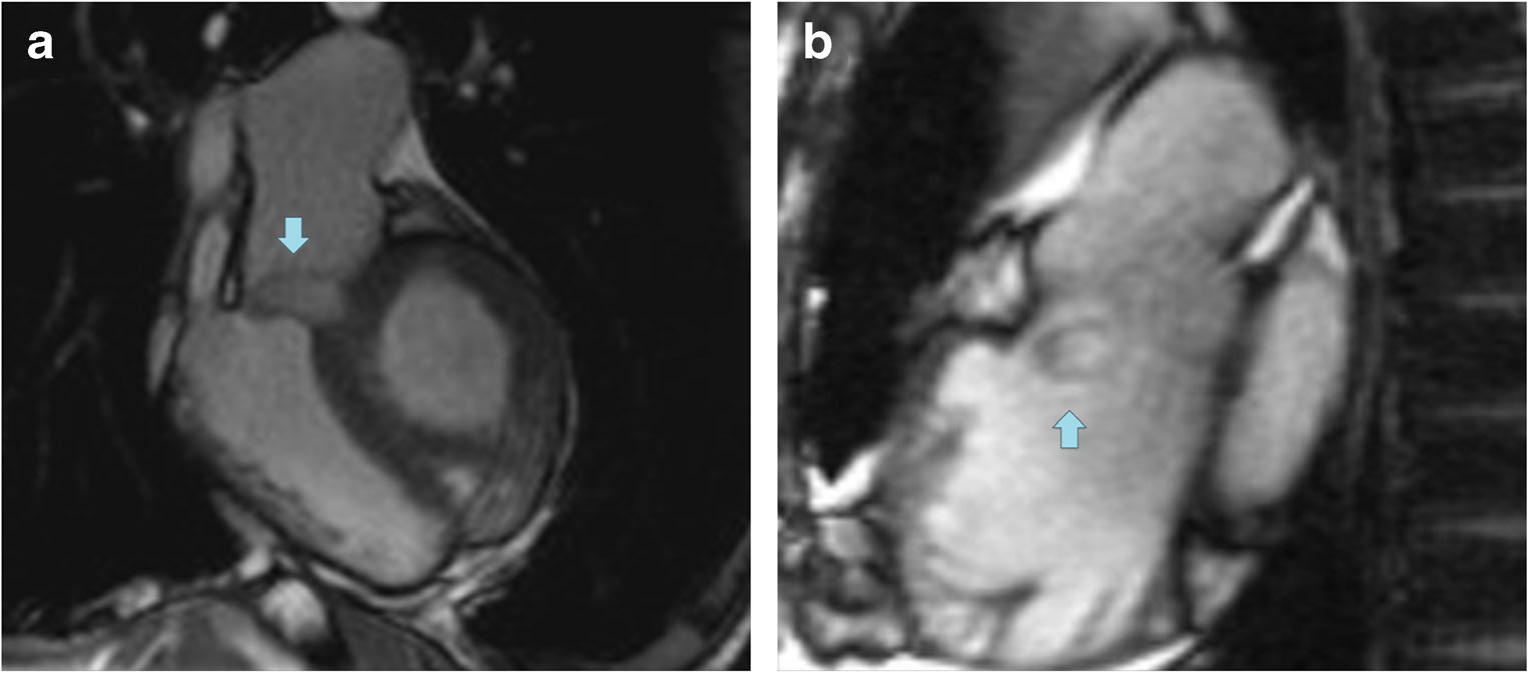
13-year-old boy with corrected TGA and EF 40 %. MRI (bSSFP sequences) in a coronal oblique projection (a) shows a finger-like projection of the IVMS (*arrows*) into the RVOT; in the sagittal oblique projection (b) through the RV shows the round tip of the IVMS aneurysm in the RV cavity
